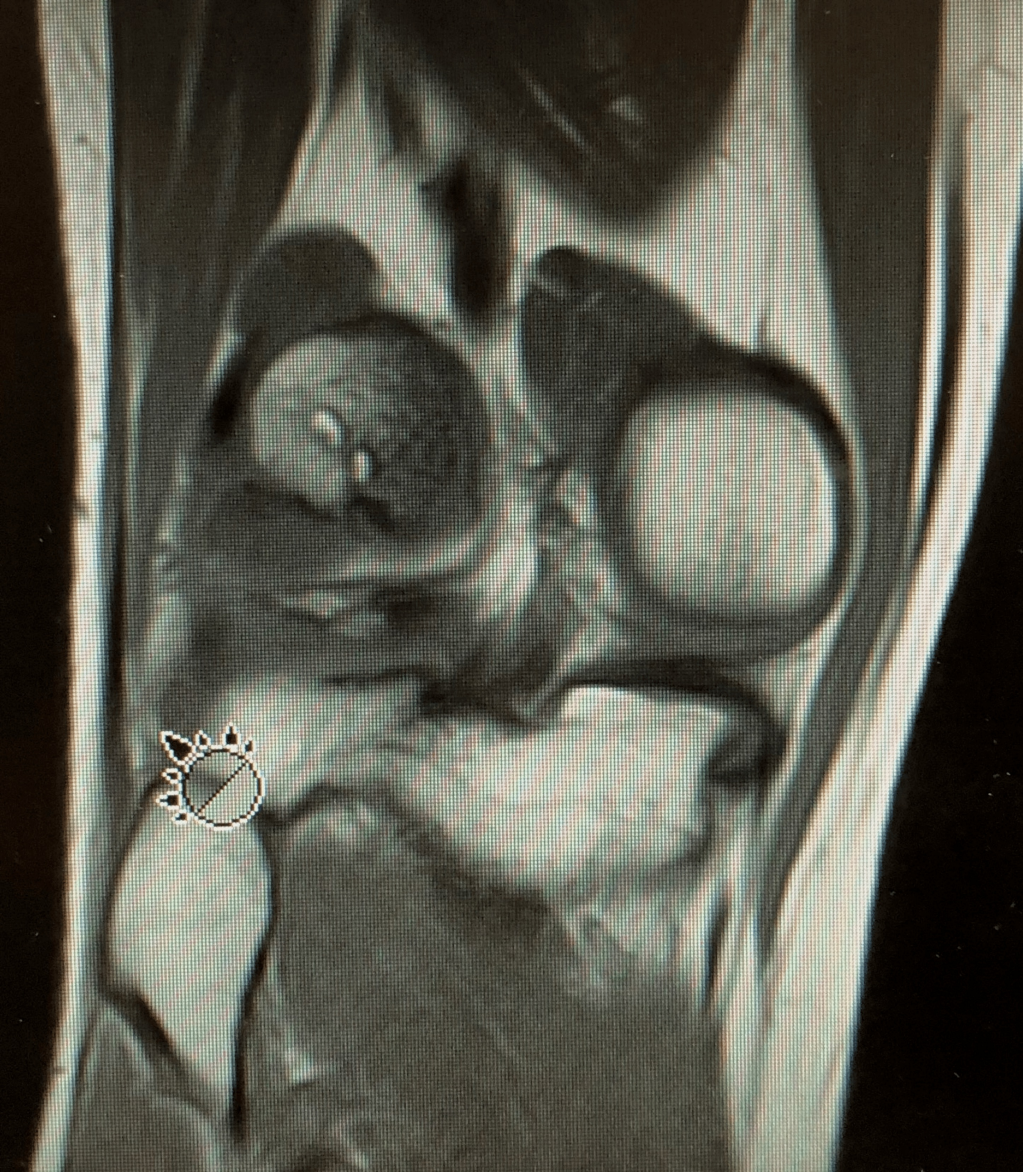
Coronal MRI of the diseased posterior lateral femoral condyle. The cursor is identifying the proximal fibular head for reference. MRI: magnetic resonance imaging
